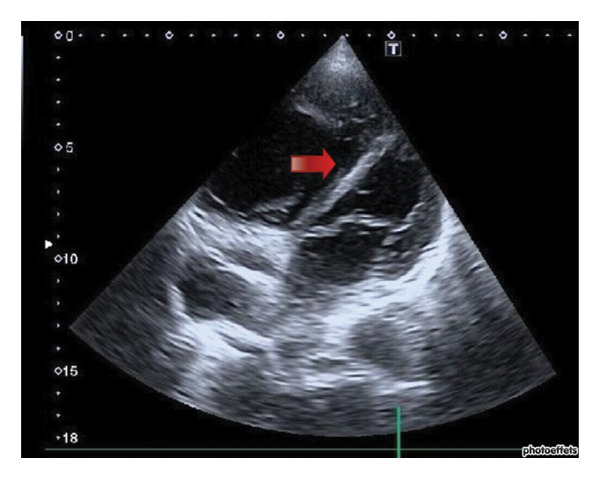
Apical four‐chamber view showing severe right ventricular dilation and septal flattening (“D‐sign”). The digital arrow indicates the flattened interventricular septum.
